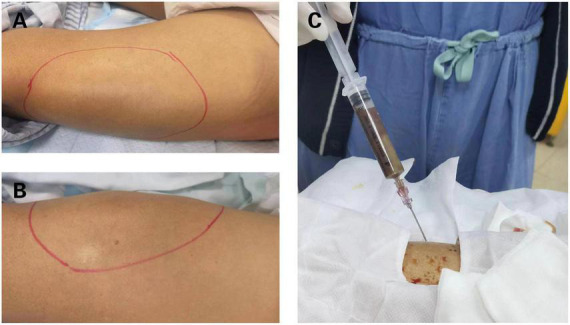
Clinical manifestation of bilateral thighs abscesses. (A) Bulging mass on the right thigh. (B) Bulging mass on the left thigh. (C) Brown fetid pus was aspirated by computed tomography (CT)-guided percutaneous puncture in right thigh abscess.
